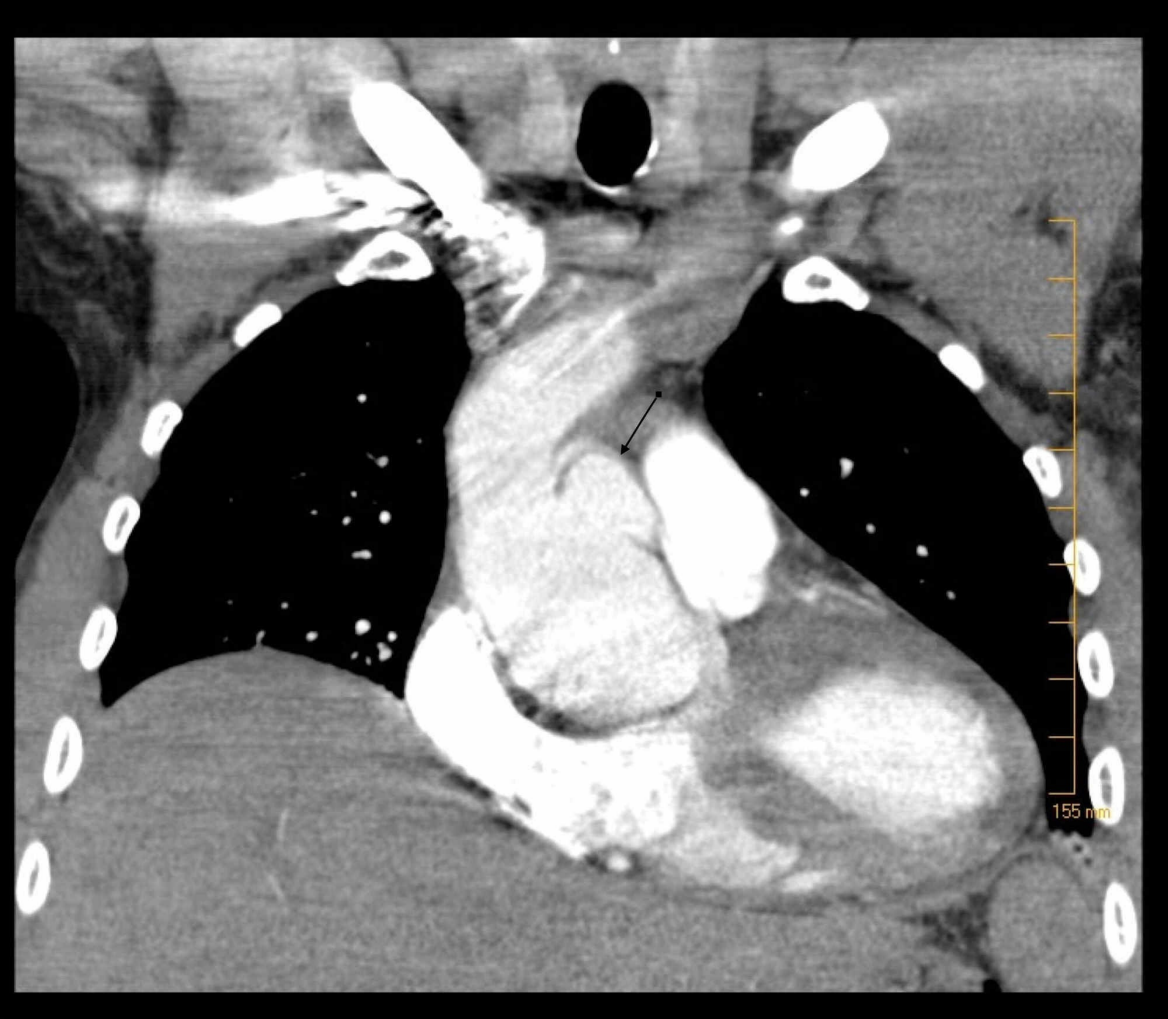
Computed tomography (CT) of the chest (coronal view); arrow showing 3 x 2 cm aneurysmal dilatation of the left sinus of Valsalva
